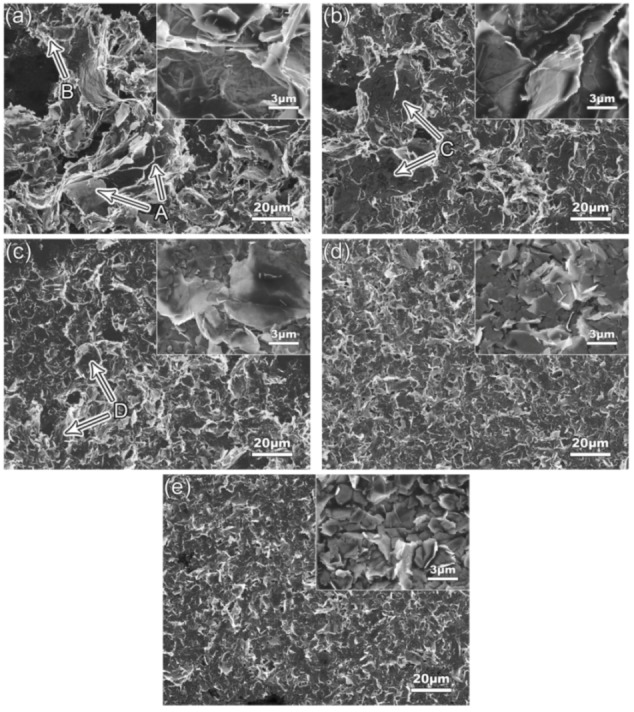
SEM images of GNPs ultrasonically dispersed for (a) 1 h, (b)2 h, (c) 3 h, (d) 4 h, (e) 5 h. Inserts are the corresponding high-magnification images.
